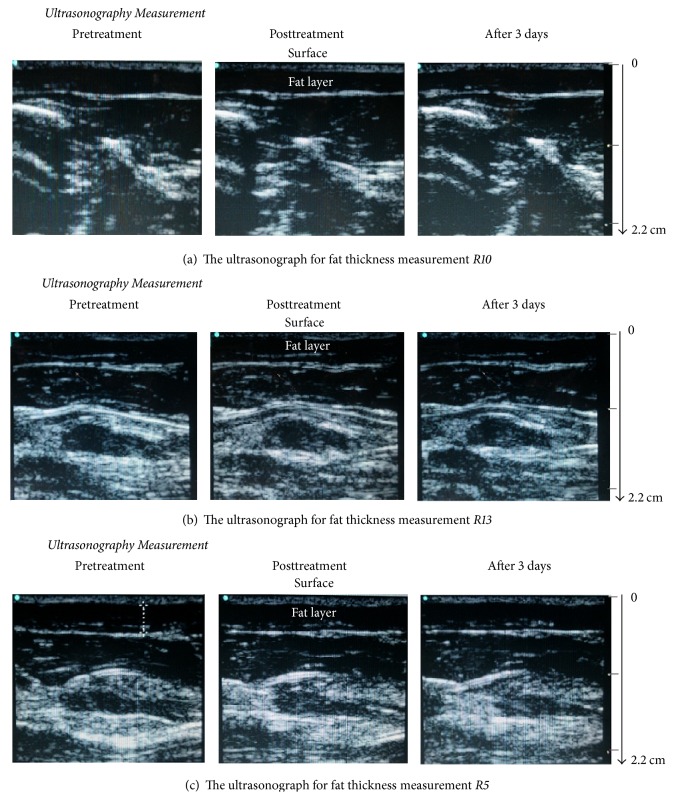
Pretreatment, posttreatment, and follow-up ultrasound images of three of the subjects (R8, R11, and R3) receiving ultrasound induced lipolysis treatment. The site of measurement was referenced to the identified anatomical trademark. (a) Fat layer showed a thickness reduction of 0.9 mm after treatment (high effect); (b) fat layer showed a thickness reduction of 0.4 mm after treatment (low effect); (c) fat layer showed a thickness reduction of 0.7 mm after treatment (Moderate effect).
